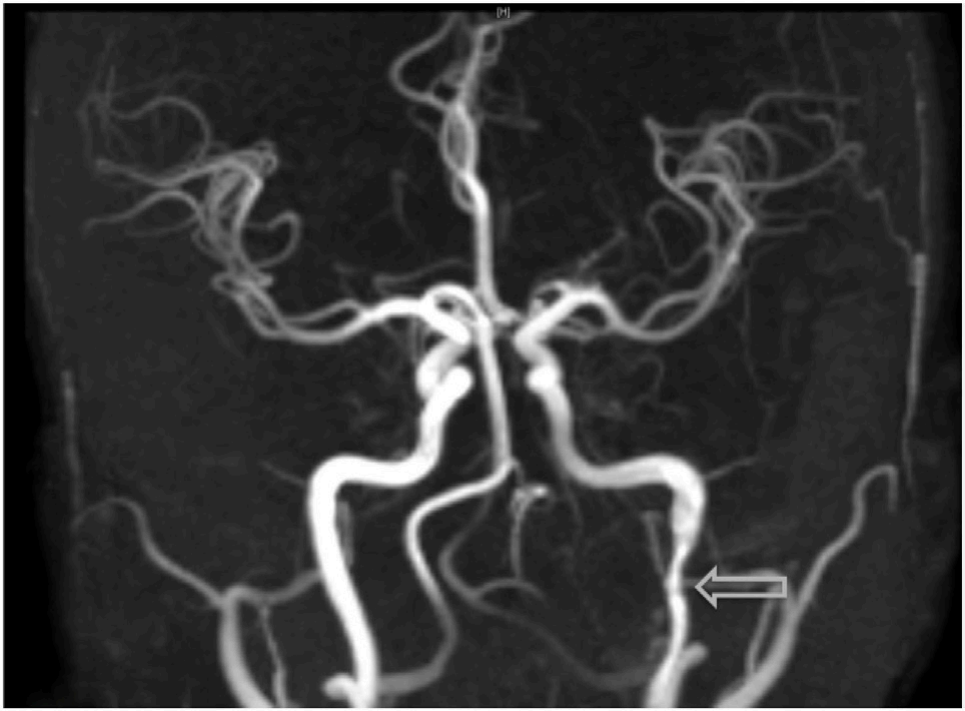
Visualization of an additional dissection noted within the left-internal carotid artery on MRI angiography, an examination which was prompted due to the onset of acute headaches.
